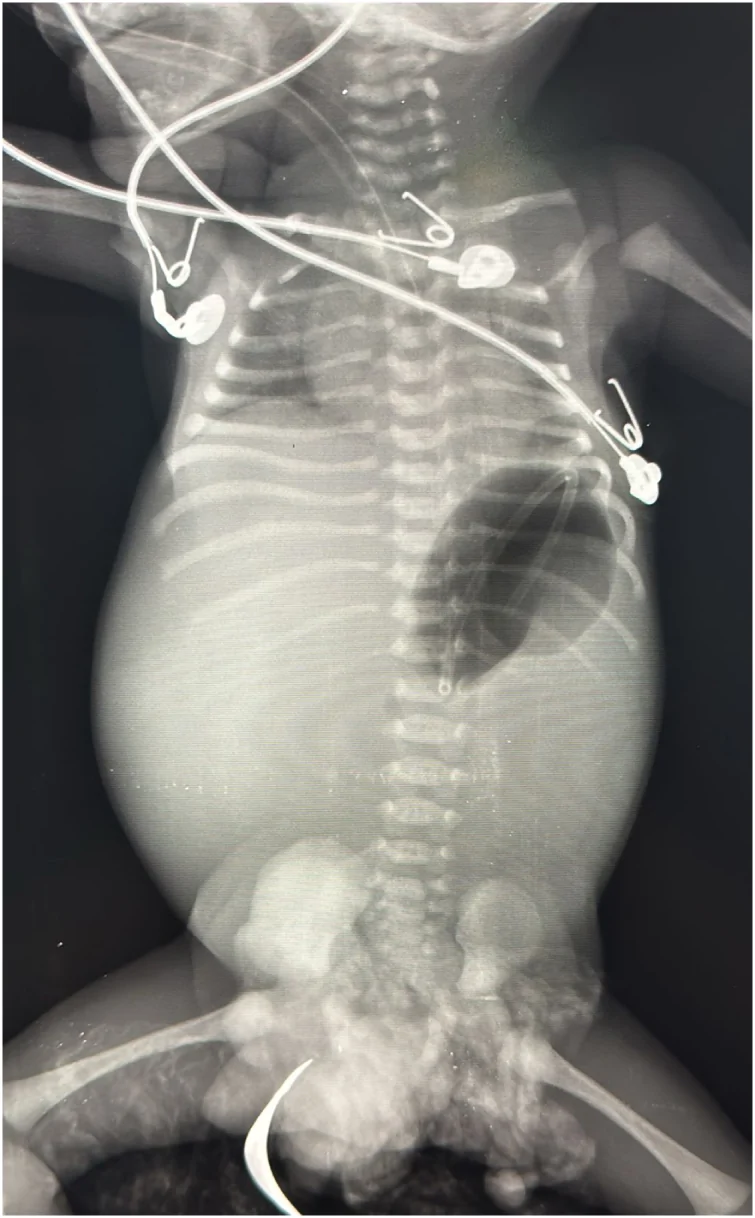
Portable abdominal radiograph demonstrating a gasless abdomen with a single gastric bubble, suggestive of intestinal atresia.
